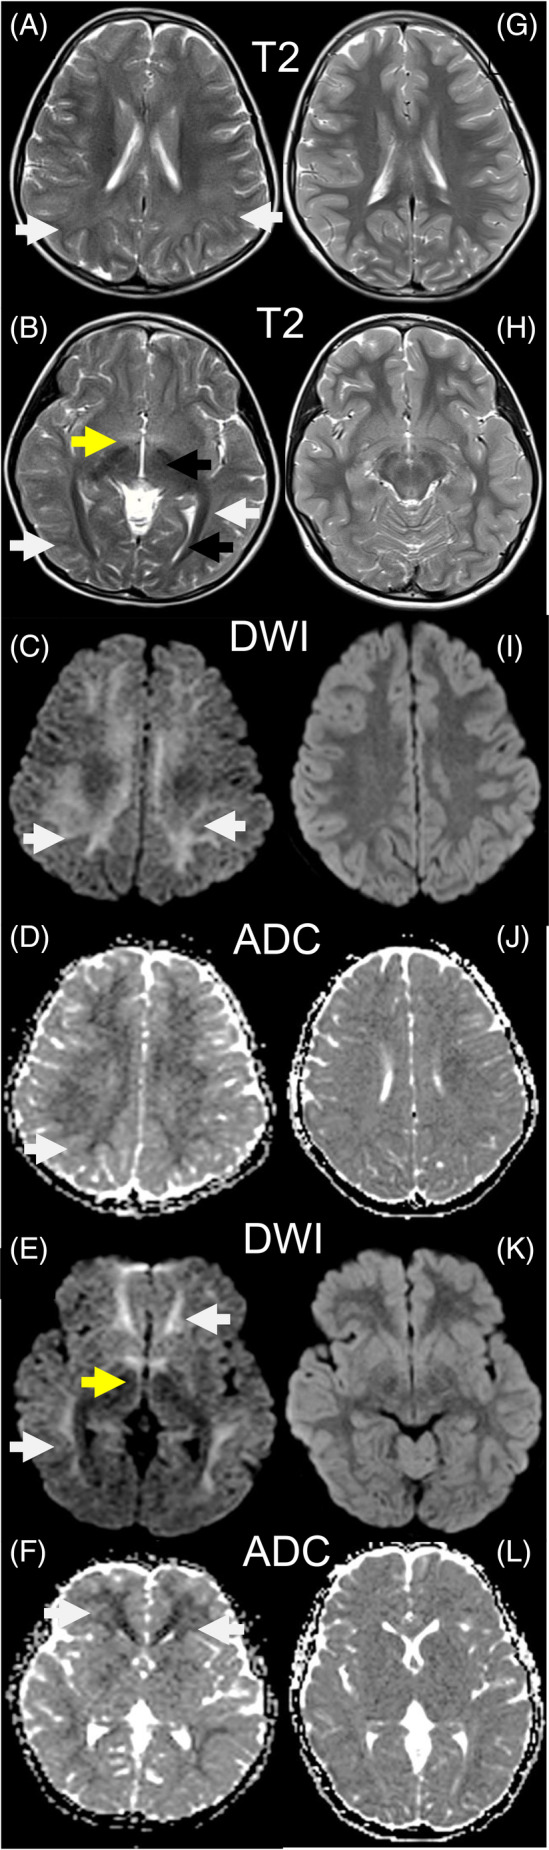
Neuroimaging of patient 1. (A–F) A brain MRI from patient one performed at the age of 6.5 years before commencement of methionine‐restricted diet shows extensive and confluent hypersignal changes of deep white matter involving all the lobes (A, B; white arrows), and the anterior commissure is also engaged (B; yellow arrow) in T2 sequences. There is corresponding diffusion restriction on diffusion‐weighted imaging (DWI) (C, D) and apparent diffusion coefficient (ADC) (E, F) sequences. The optic radiation and internal capsule are spared (B; black arrows). (G–L) The brain MRI at 7.5 years (after 12 months of methionine‐restricted diet) shows resolution of all white matter changes without residual volume loss or signal change.
